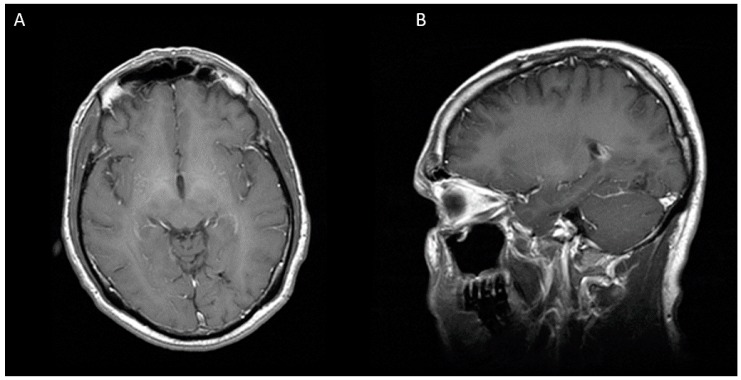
Eight months after second stereotactic needle aspiration, MRI is showing a complete resolution of cerebral abscess. (A) Axial view (B) Sagittal view.
